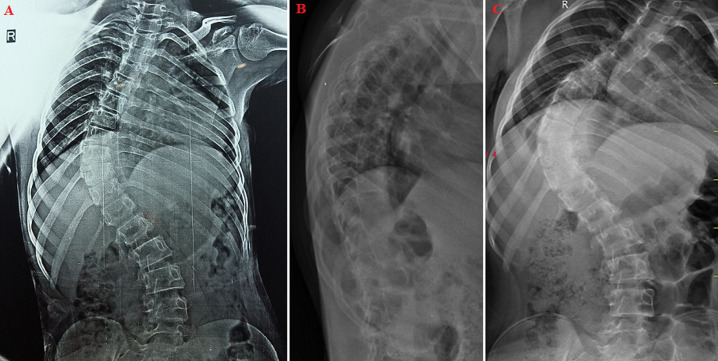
A, B, C) X-ray presentation of kyphoscoliosis
